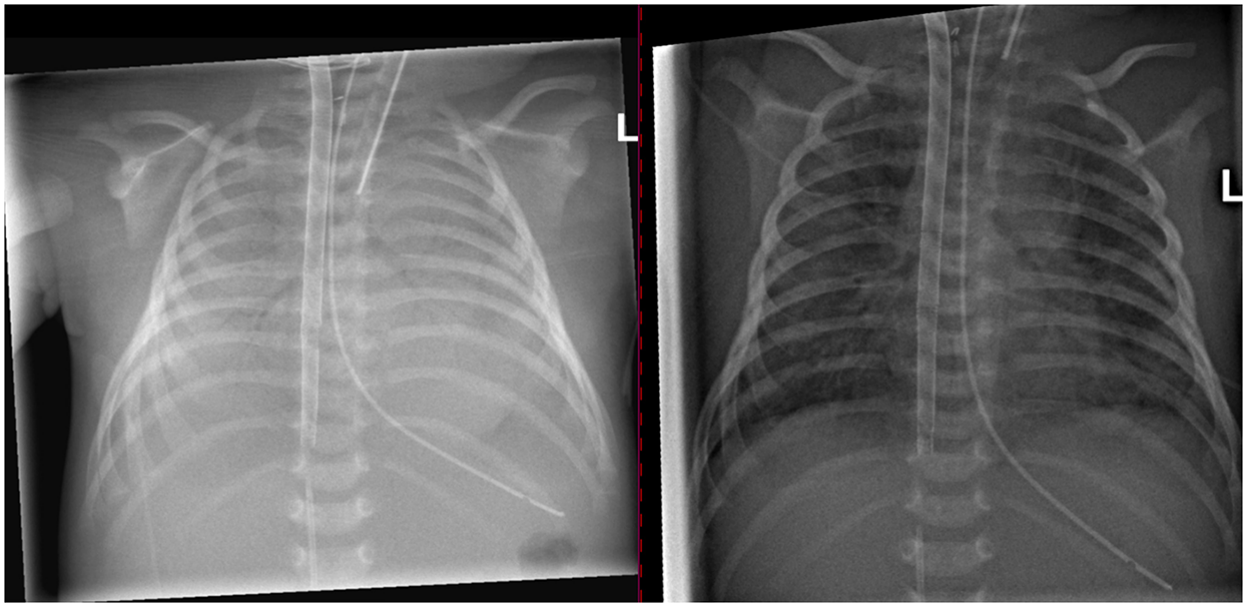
Chest x-ray prior to the recruitment maneuver on the left side and after recruitment on the right side.
